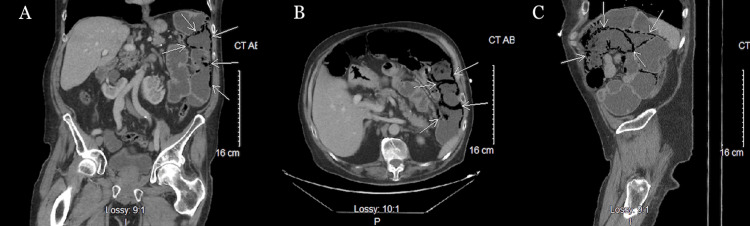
CT scan showing PCI in the left upper quadrant of the abdomen. A) Coronal view. B) Axial view. C) Sagittal view. This is an original CT scan of the patient detailed by this report. Pathology indicated by white arrows. PCI: pneumatosis cystoides intestinalis.
